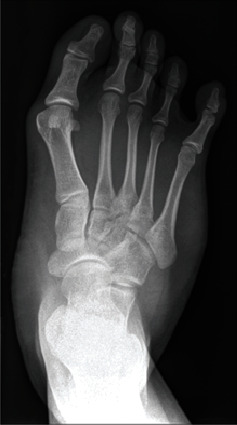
Preoperative anteroposterior radiograph.
